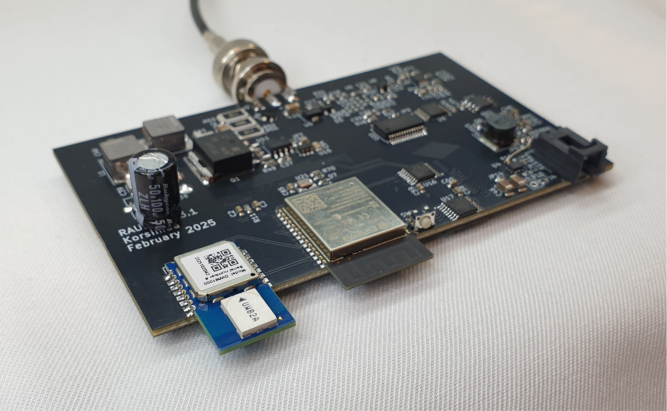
An ultrasound sensor unit with the UWB module installed. Wireless triggering enables multiple sensors to be used as a coordinated network for structural health monitoring. The UWB module is easy to integrate into existing designs due to its small footprint (<210 mm${}^{2}$).
